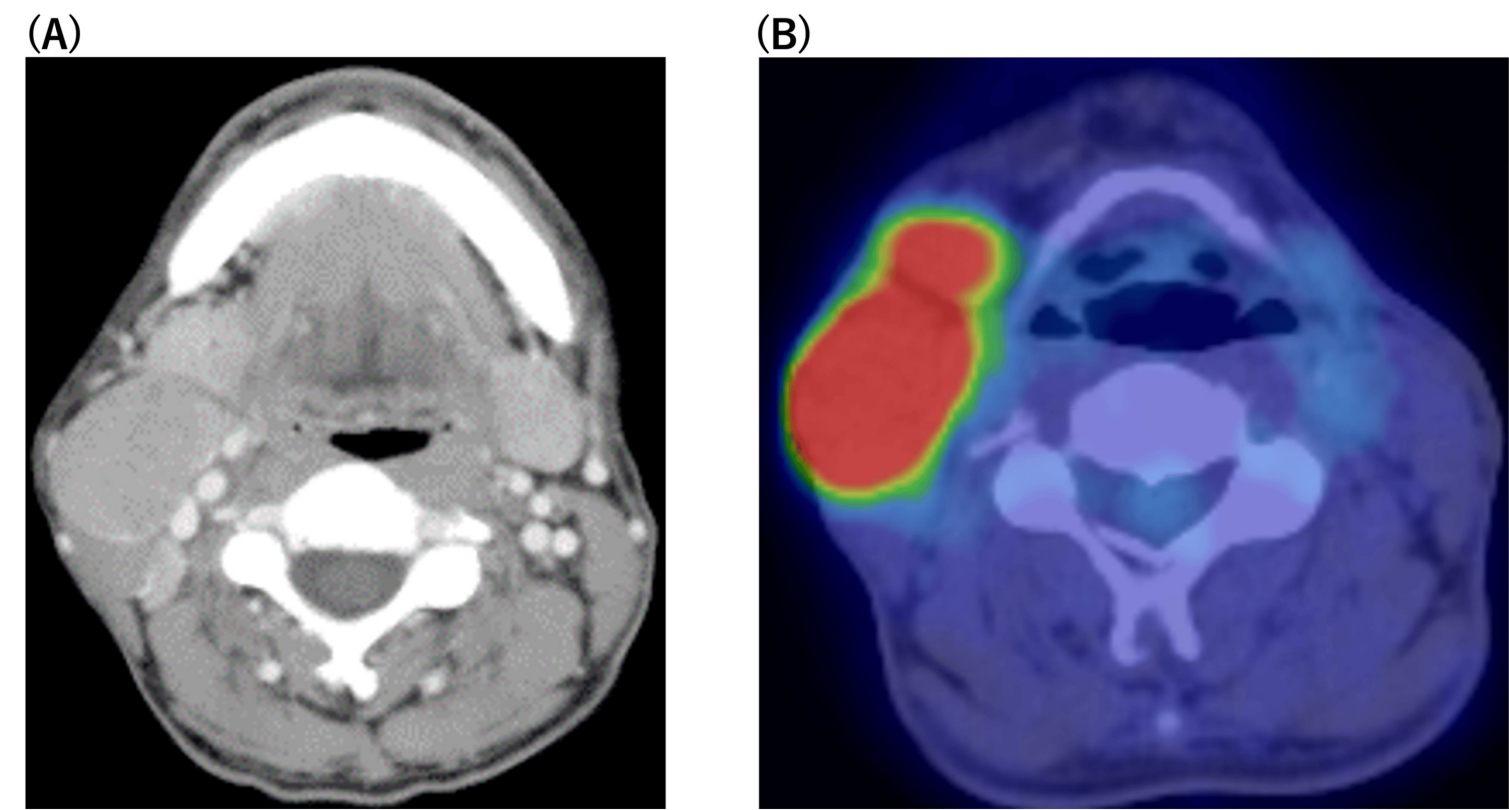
Pre-therapeutic findings. (A) Neck contrast-enhanced CT image showing swelling in the right submandibular gland with lymphadenopathy; (B) 18F fluorodeoxyglucose (FDG) PET-CT image showing high uptake of FDG in the right submandibular gland and lymph nodes.
